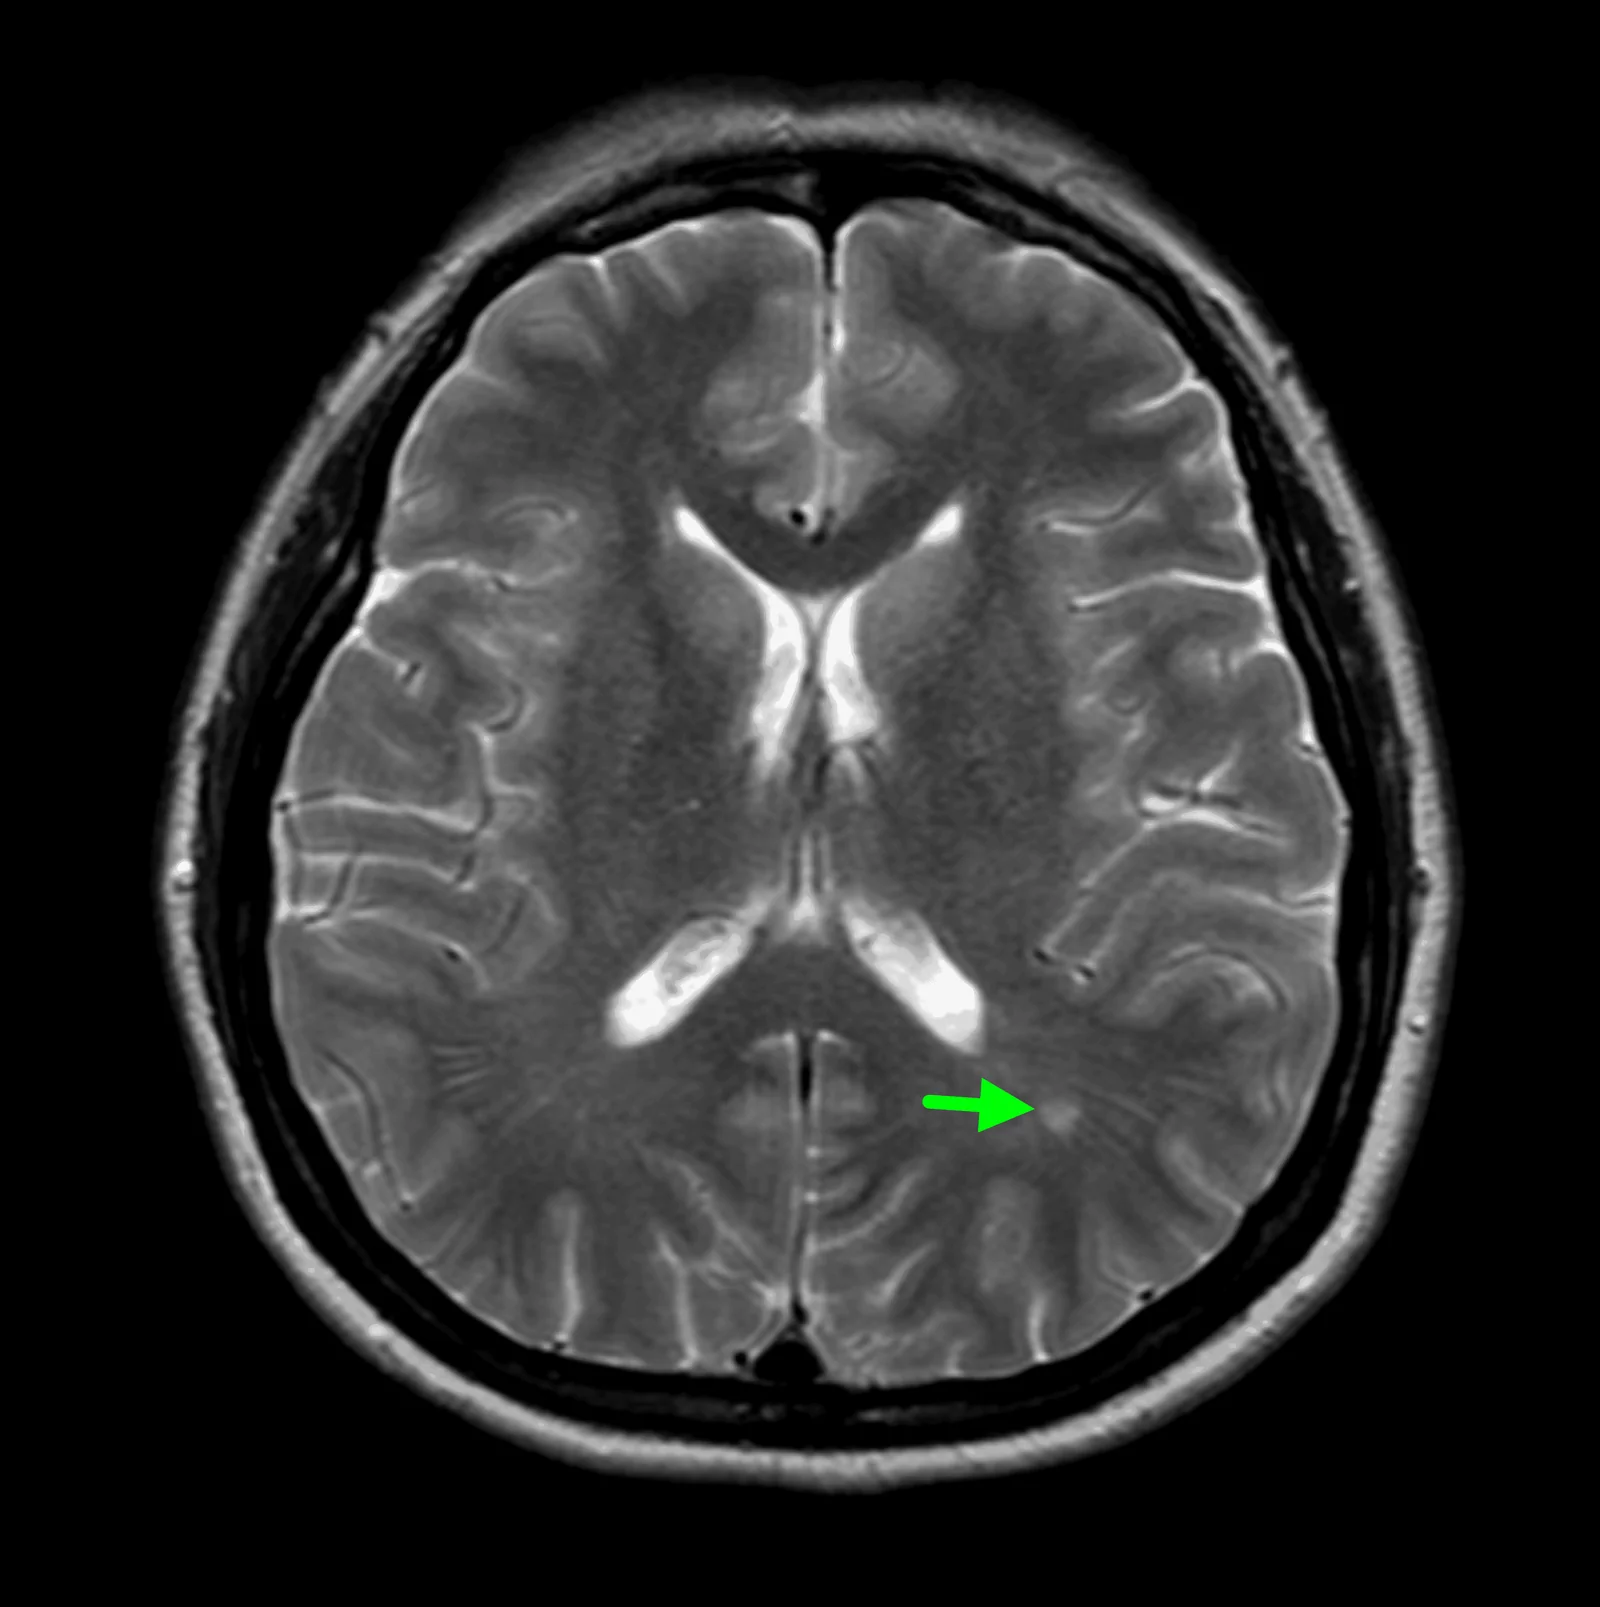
Outside hospital T2 MRI brain showing a single small left-sided white matter lesion (day 77 post initial COVID-19 vaccination) This solitary lesion was initially non-specific but, in retrospect, may represent early preclinical demyelination.
